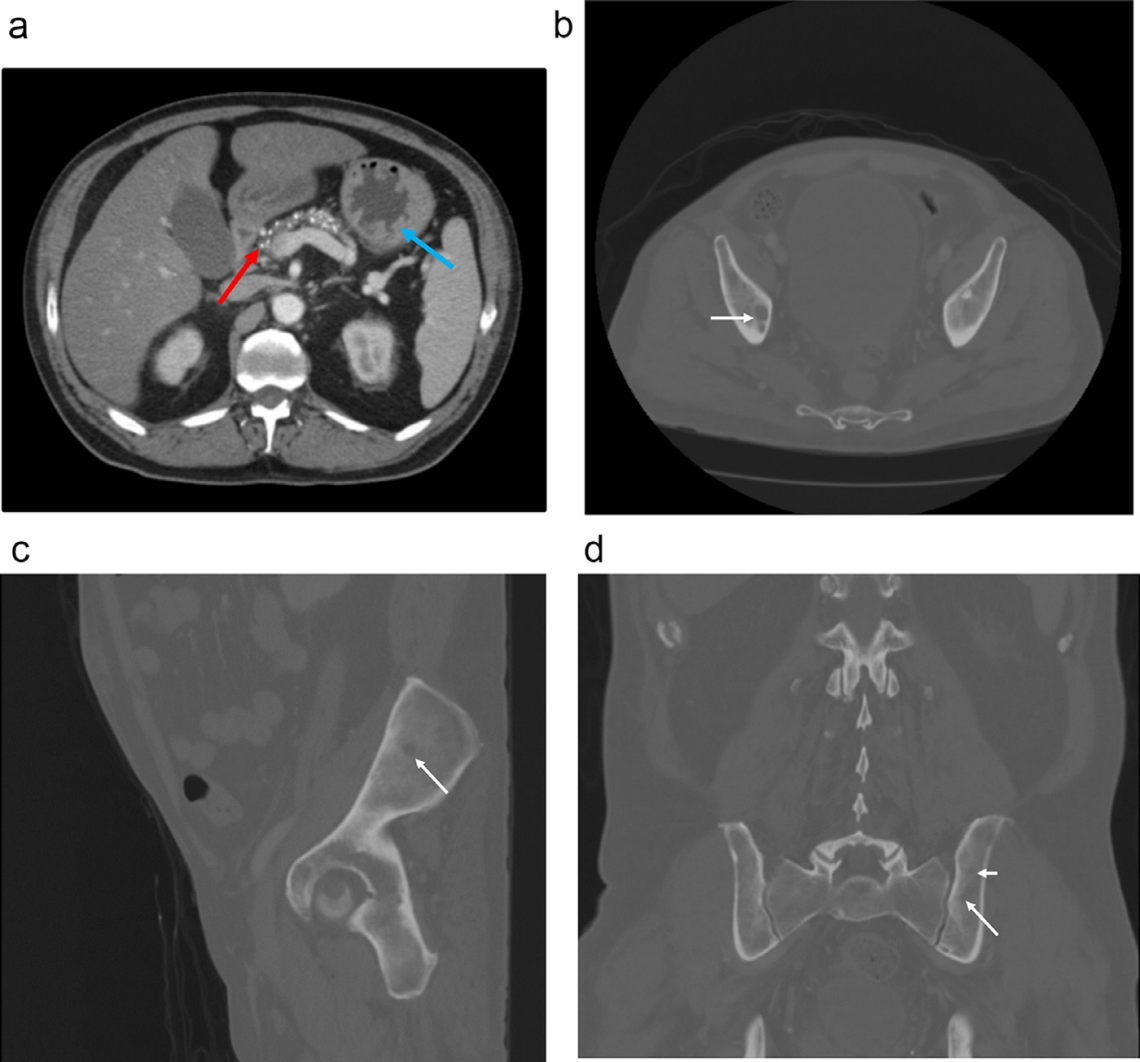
Computed tomography of the abdomen and pelvis notable for acute diverticulitis of descending colon with abscess (A, blue arrow), liver parenchymal disease, chronic pancreatitis (A, red arrow), and incidental lytic lesions (B-D, white arrows).
